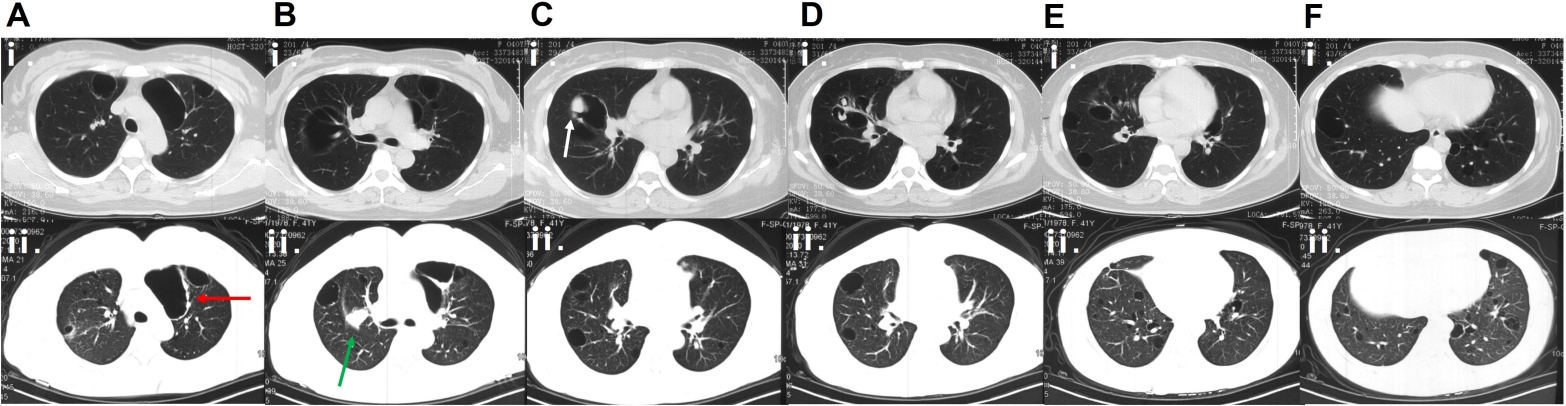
CT scans at presentation and during follow-up. (Ai–Fi) Chest CT scan without contrast in November 2018, showing multiple cysts in both lungs, with a solid nodule (indicated by the white arrow) observed within the cyst in the middle lobe of the right lung. (Aii–Fii) Chest CT scan with contrast in April 2020 showing multiple air-containing cavities in both lungs, with wall thickening and small nodules (indicated by the red arrow) developing in the left upper lobe, and a nodule (indicated by the green arrow) adjacent to the mediastinum in the right lung.
